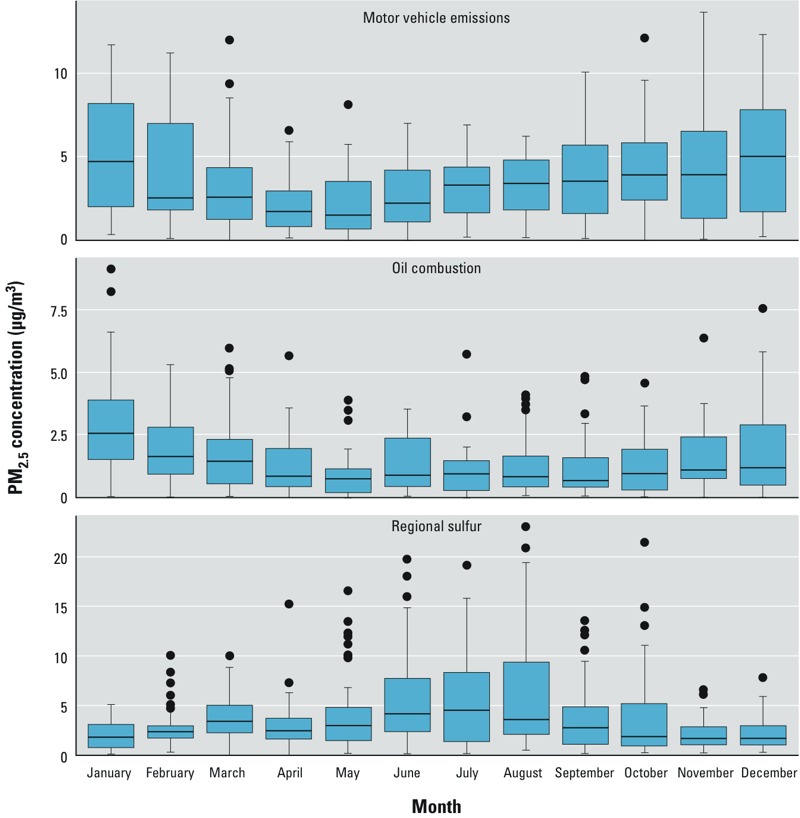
PM_2.5_ concentrations attributable to motor vehicle emissions, oil combustion, and regional sulfur, by calendar month in Hartford, CT. Boxes extend from the 25th to the 75th percentile, horizontal bars represent the median, whiskers extend 1.5 times the length of the IQR above and below the 75th and 25th percentiles, respectively, and outliers are represented as points. The numbers of samples in each month were as follows: January, 37; February, 41; March, 48; April, 49; May, 50; June, 43; July, 40; August, 50; September, 51; October, 47; November, 38; December, 39.
